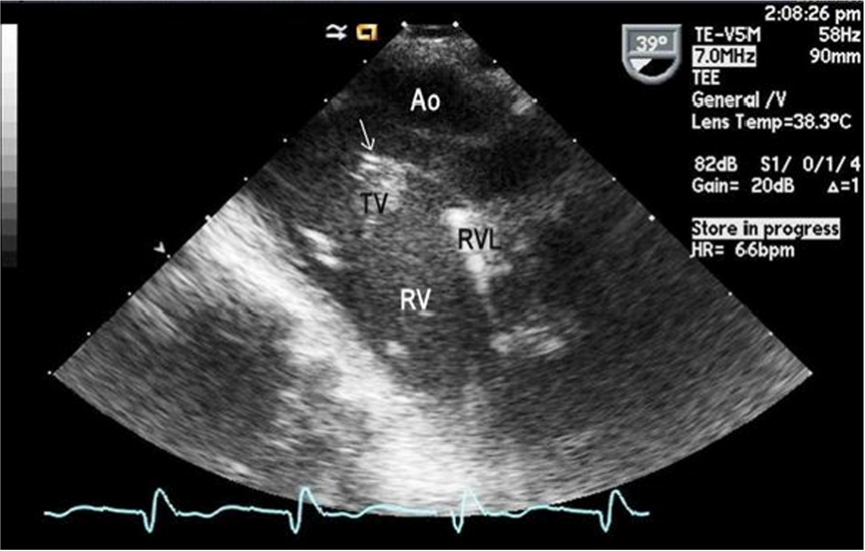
Transesophageal echocardiogram showing a 1.2 × 0.7 cm vegetation (arrow) attached to the right ventricular pacemaker lead in the right atrium at the coaptation of the tricuspid valve leaflets. Ao = Aorta, TV = Tricuspid Valve, RVL = Right Ventricular Lead, RV = Right Ventricle.
